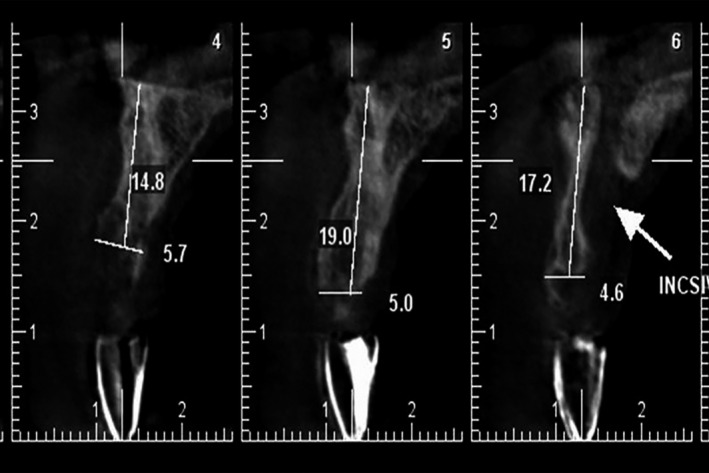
CBCT with radiographic stent
